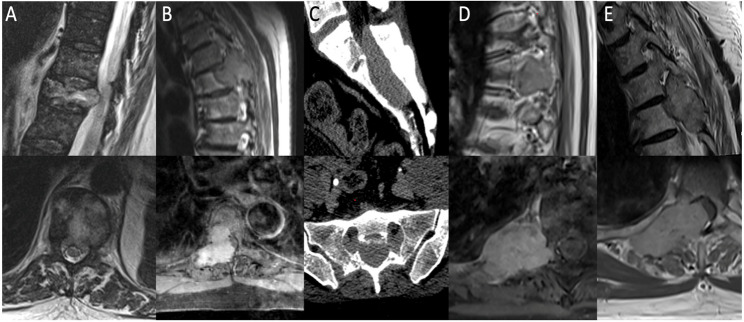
Preoperative imaging of patients #1–#5 (A–E). For each patient, the upper panel shows a sagittal view and the lower panel an axial view. MRI was performed in all patients except patient #3, who underwent only CT imaging. A Patient #1: vertebral metastasis with pathologic fracture of T10 and epidural extension into the spinal canal. B Patient #2: mass lesion arising from the right pedicle of T6, extending toward the right ribs and into the spinal canal. C Patient #3: predominantly intraspinal mass lesion at S1–S3. D Patient #4: predominantly paraspinal mass lesion involving the right pedicle of T7 and the neural foramina without spinal canal involvement. E Patient #5: intra- and extraspinal mass lesion involving T3 and T4 on the right side
